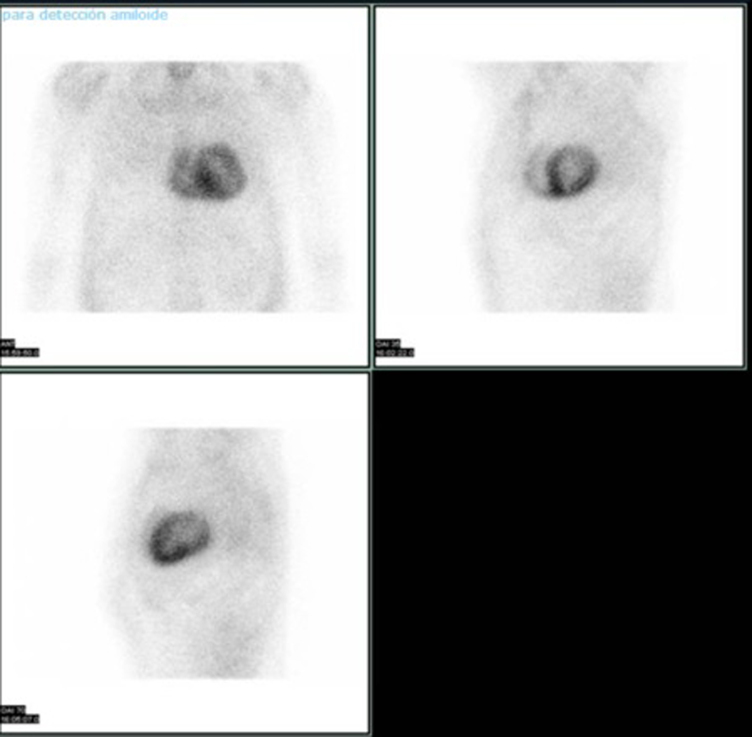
Bone scintigraphy with 99mTc-DPD demonstrating Grade 3 myocardial uptake (Perugini classification).
